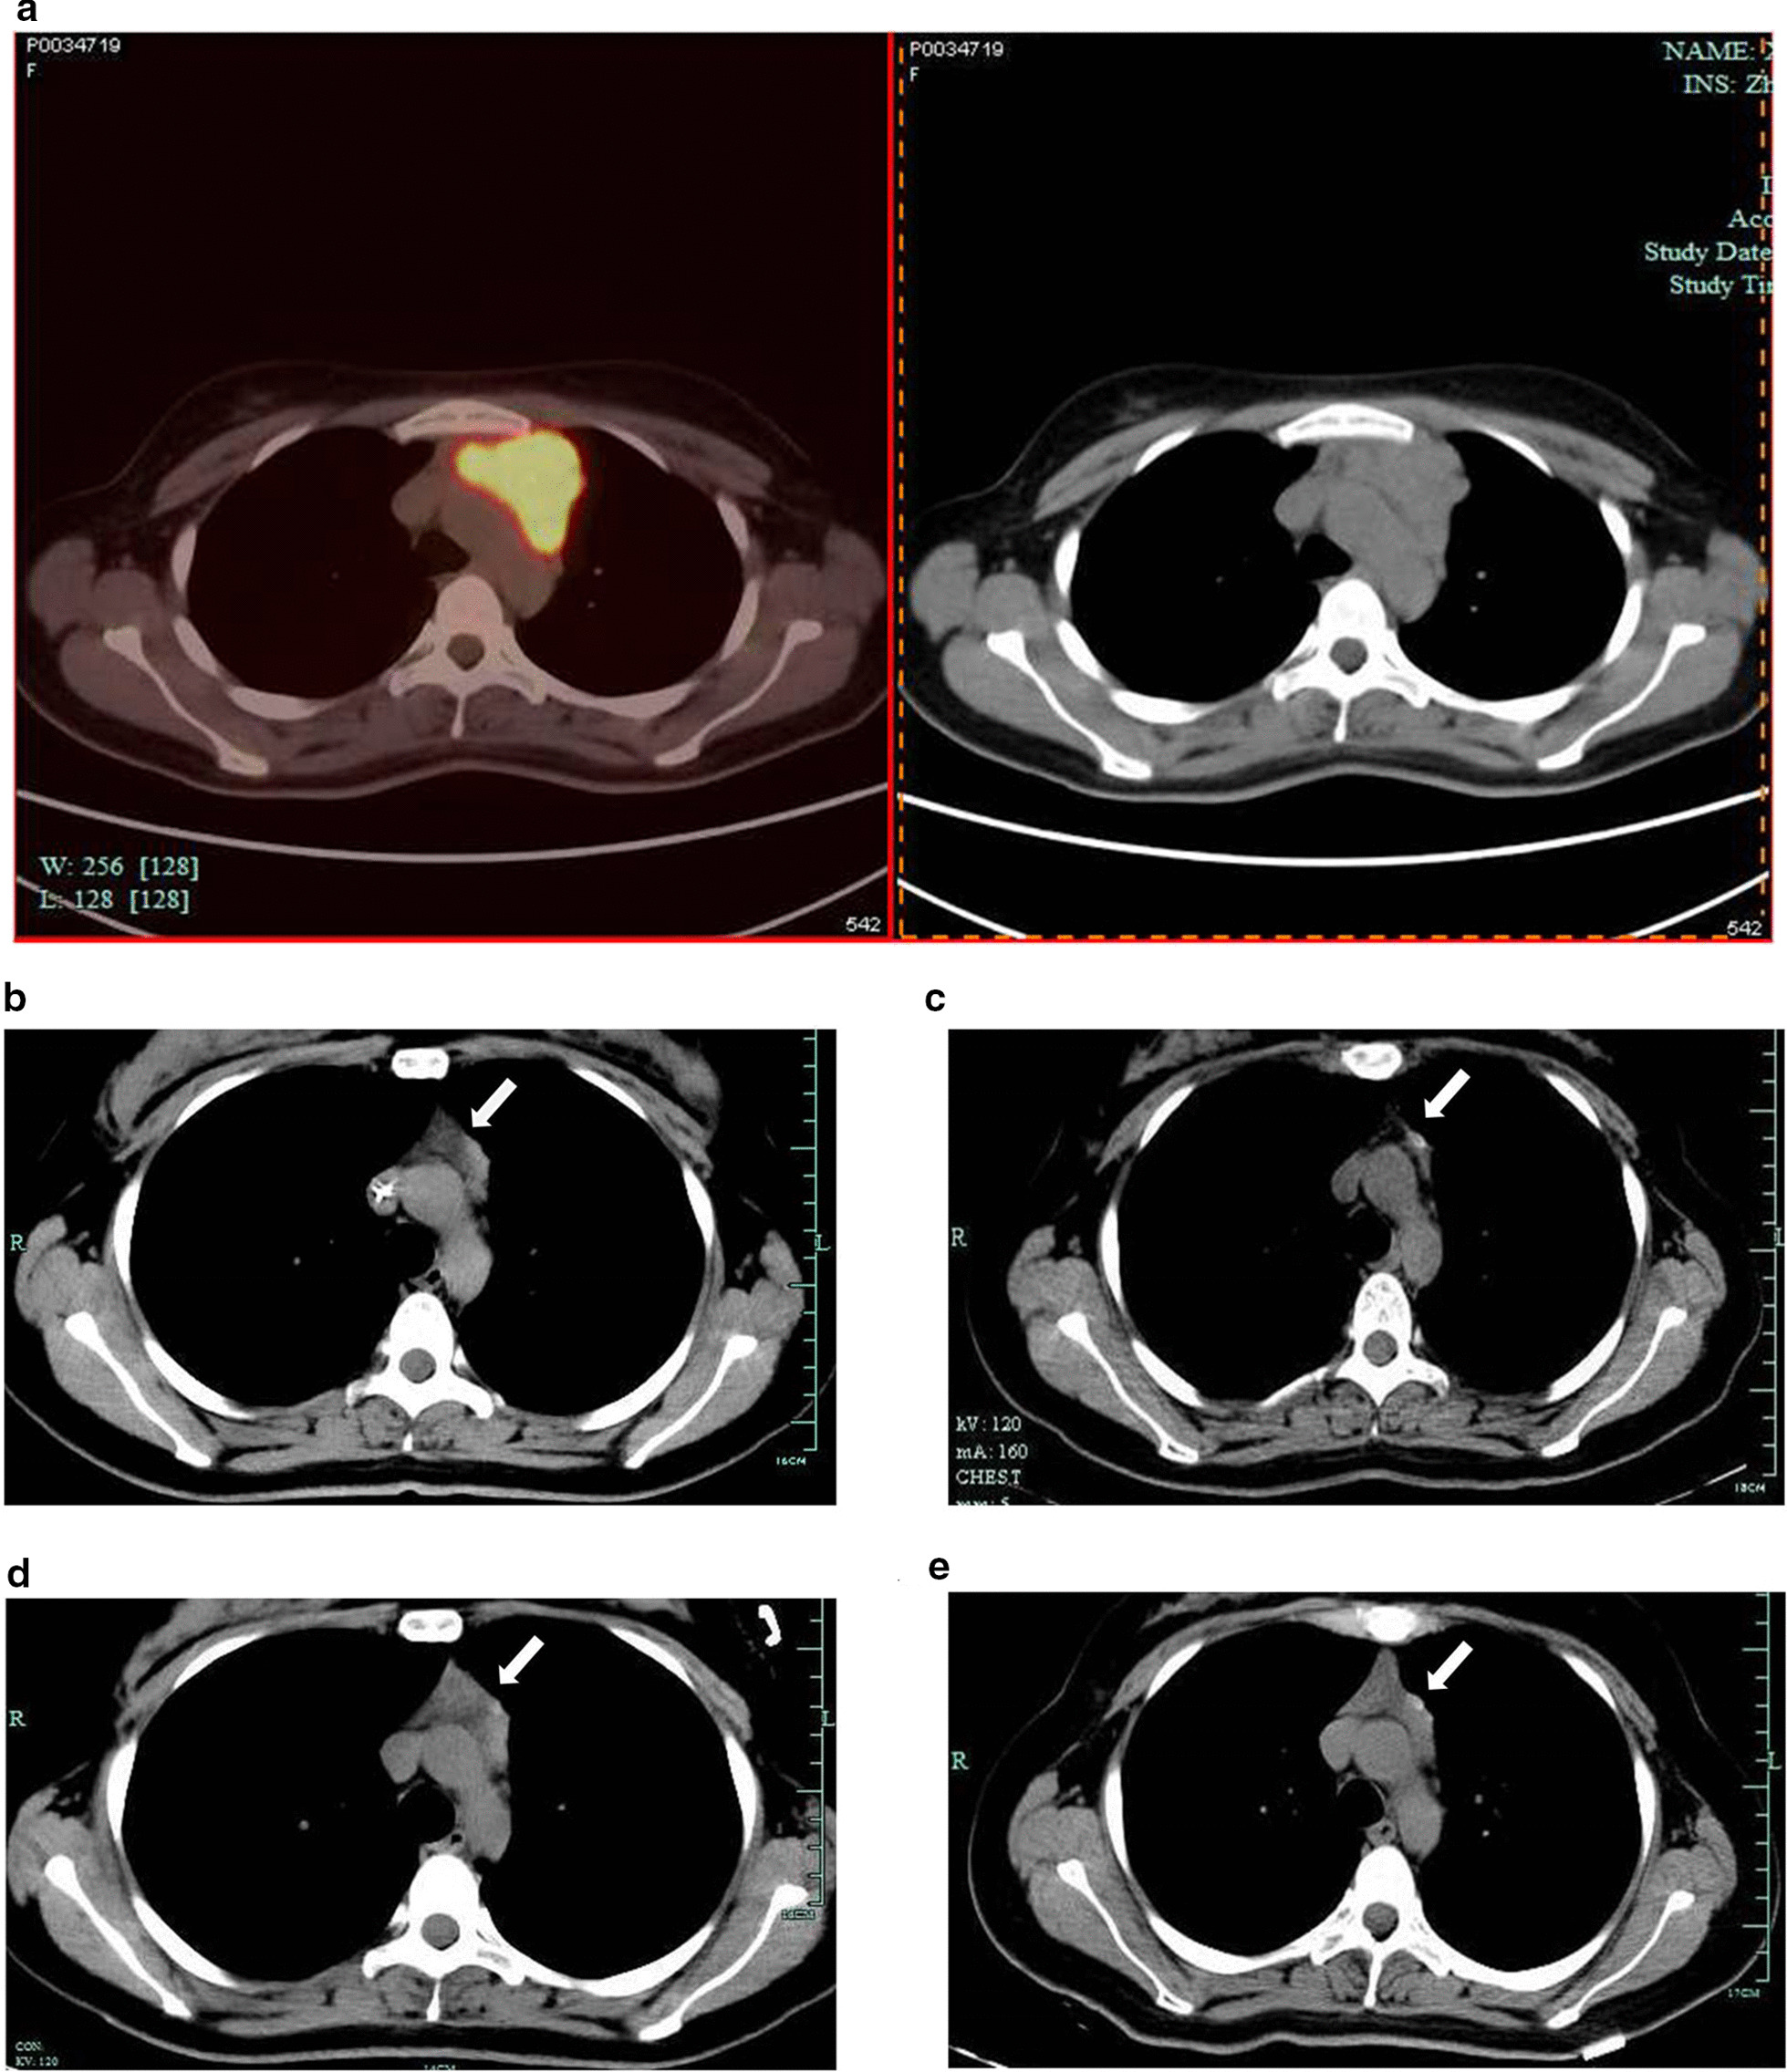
Representative PET/CT or CT images of the superior mediastinal mass in the patient’s chest during treatment. Representative PET/CT images of the anterior mediastinal lesions before treatment, showing multiple lymphadenopathies in the left anterior superior mediastinum and adjacent to the aortic arch, with the maximum SUV of 22 (a); After 8 courses of treatment, anterior mediastinal mass could be still seen (b); 3 months after ASCT, the anterior mediastinal mass disappeared (c); 6 months after ASCT anterior mediastinal mass appeared repeatedly (d); 12 months after ASCT, the anterior mediastinal mass observed similar to that 6 months after ASCT (e)
